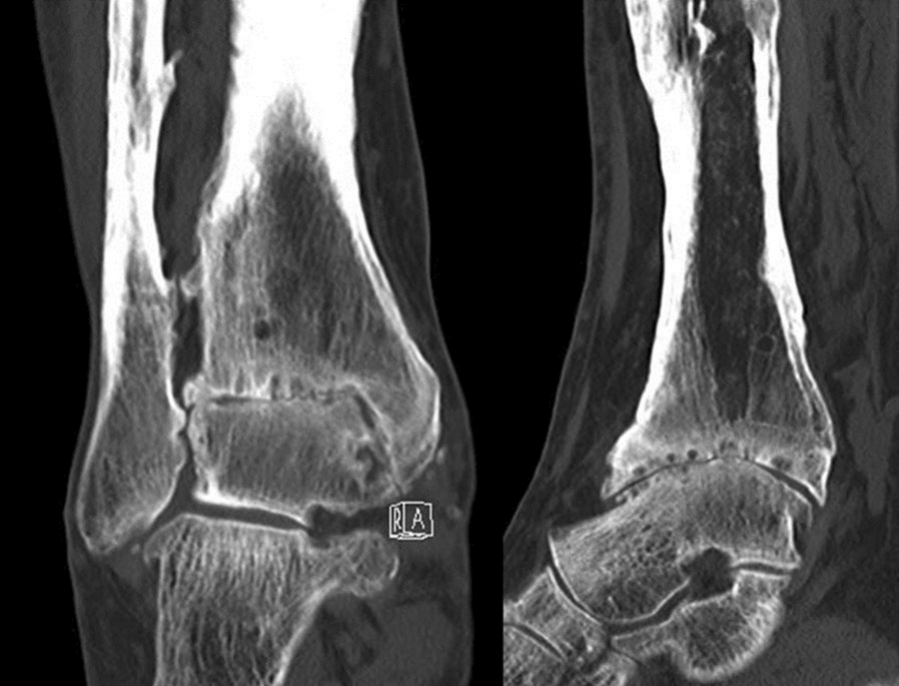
Coronal and sagittal CT sections
